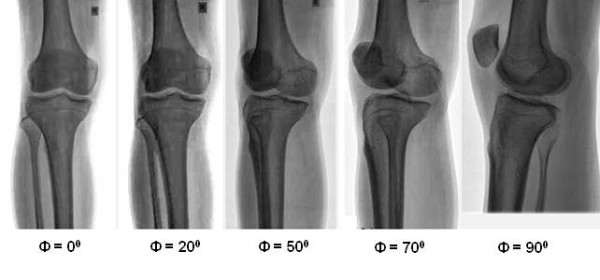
Benchmark X-rays taken atpredetermined rotation angles.
